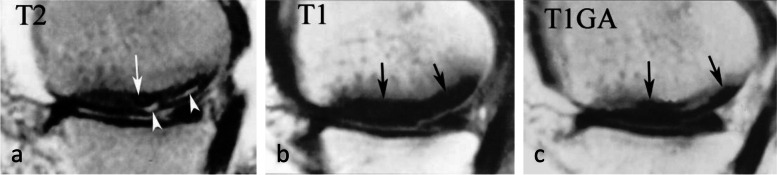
Subchondral insufficiency fracture complicated with osteonecrosis (SIF-ON) and early subchondral collapse. a T2-weighted MR image shows a wide subchondral low signal intensity area (arrow). On T1-weighted images without (b) and after contrast injection (c) a large portion of this area does not enhance after intravenous contrast injection (arrows in c). Note also a thin line with high fluid-like signal intensity in dissected subchondral fracture on T2-weighted image (arrowhead in a)
